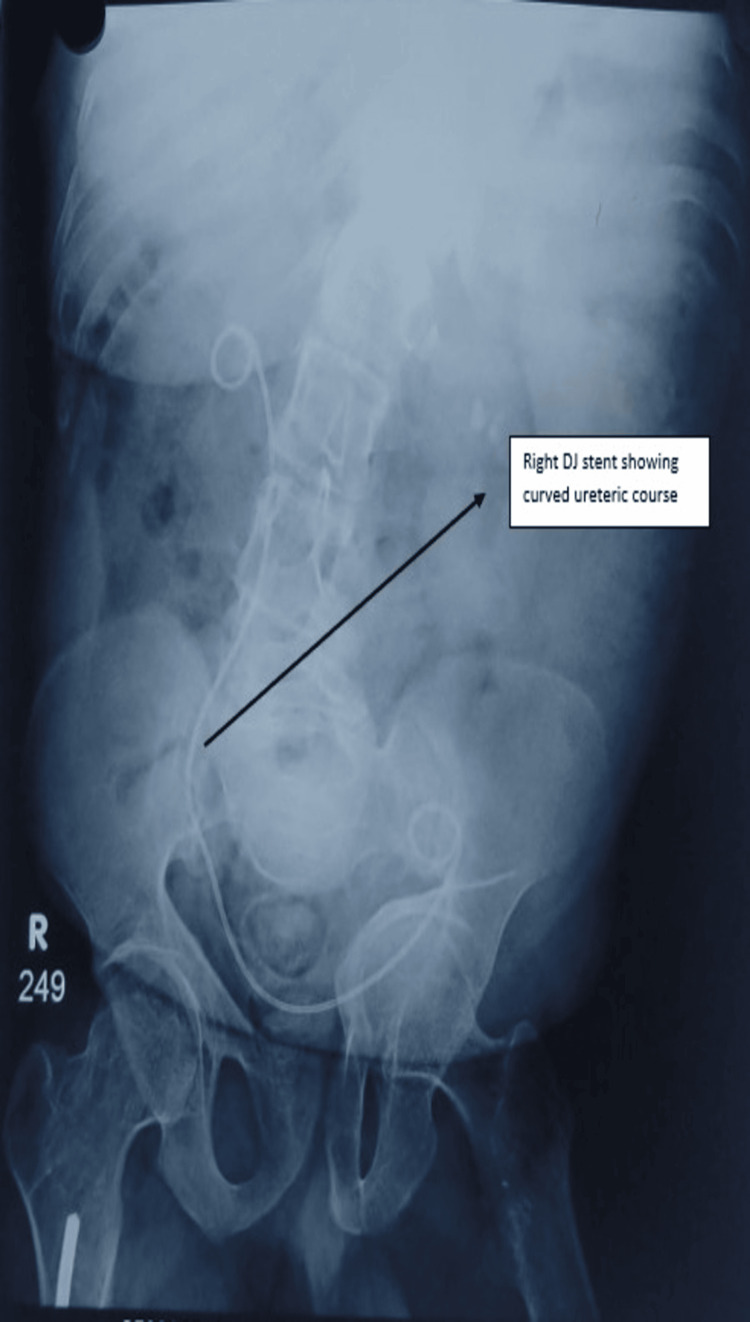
Post-procedure radiograph demonstrating double-J stent placement with marked ureteral angulation. Postoperative X-ray KUB showing stone clearance and the curved course of the right ureter. The black arrow indicates the course of the double-J stent. Plain radiograph obtained after ureteroscopy showing double-J stent placement with a nonlinear and markedly angulated ureteral course. The abnormal stent trajectory visually demonstrates the distorted ureteral anatomy encountered intraoperatively and highlights the technical difficulty involved in accessing and managing the distal ureteric calculus. KUB: Kidneys, Ureters, and Bladder.
